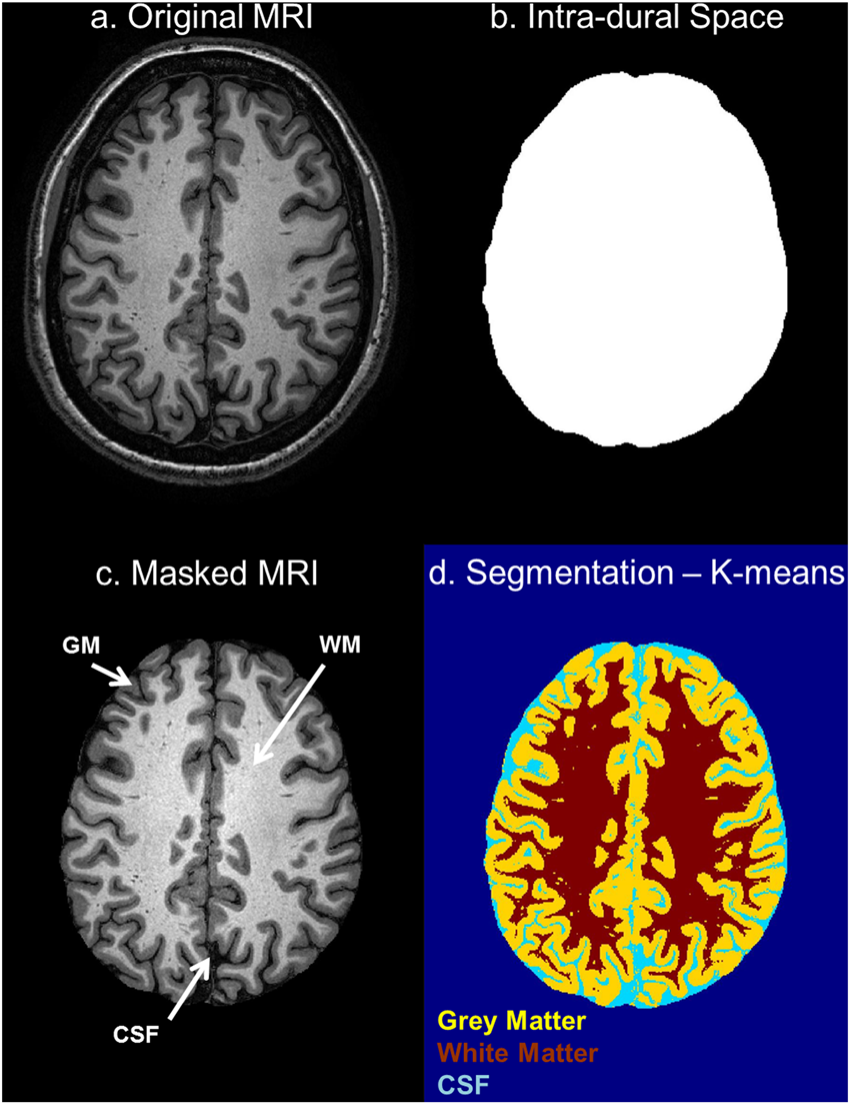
Segmentation of the cerebrum. (a) Original axial T1-weighted MRI, (b) intra-dural space obtained as the space surrounded by the previously segmented dura mater, (c) masked T1-weighted MRI, and (d) the image resulting from application of the *k*-means algorithm.
